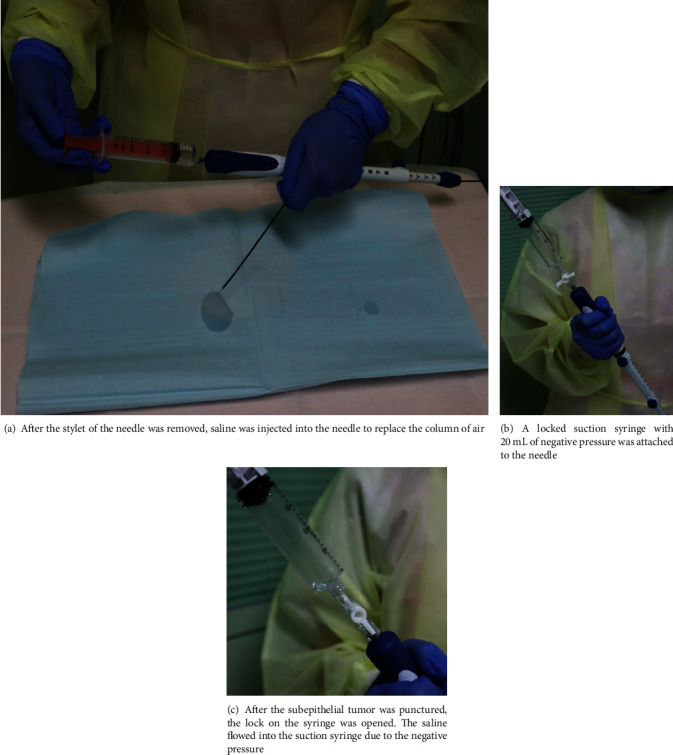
Wet technique of endoscopic ultrasonography-guided fine-needle aspiration.
